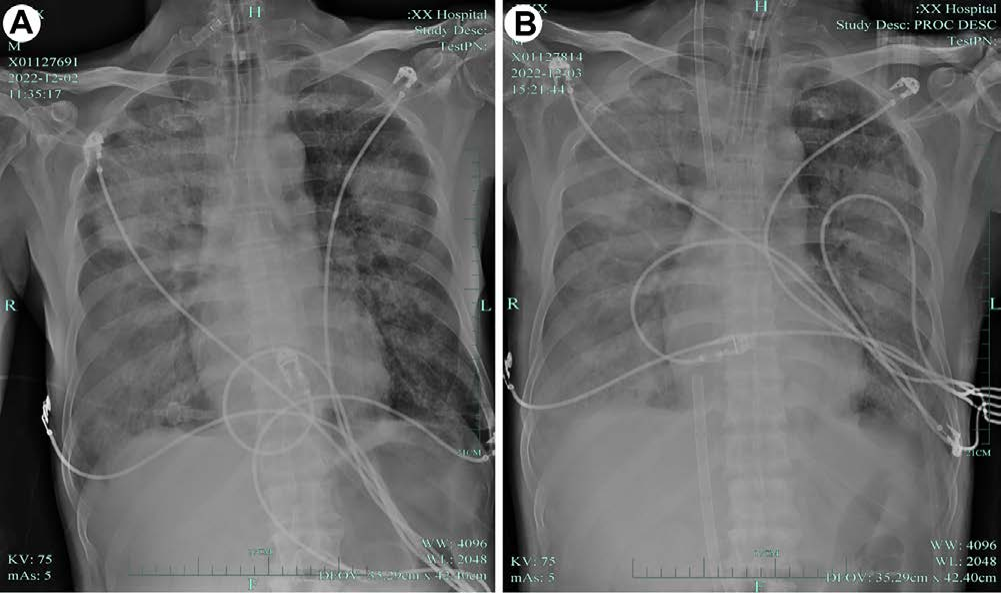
Bedside chest X-ray on December 2, 2022 (A) and December 3, 2022 (B).
